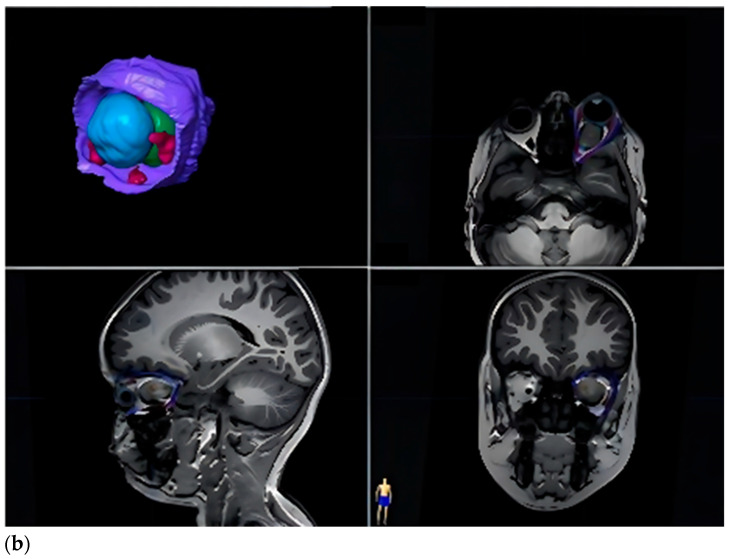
(a) Preoperative MRI-CT fusion is essential for navigating intraorbital lesions and accurately identifying soft-tissue structures (e.g., extraocular muscles, optic nerve, and tumor). (b) Constructed biomodels can be printed after this segmentation to help visualize tumor relationships, plan surgical corridors, and anticipate postoperative outcomes.
